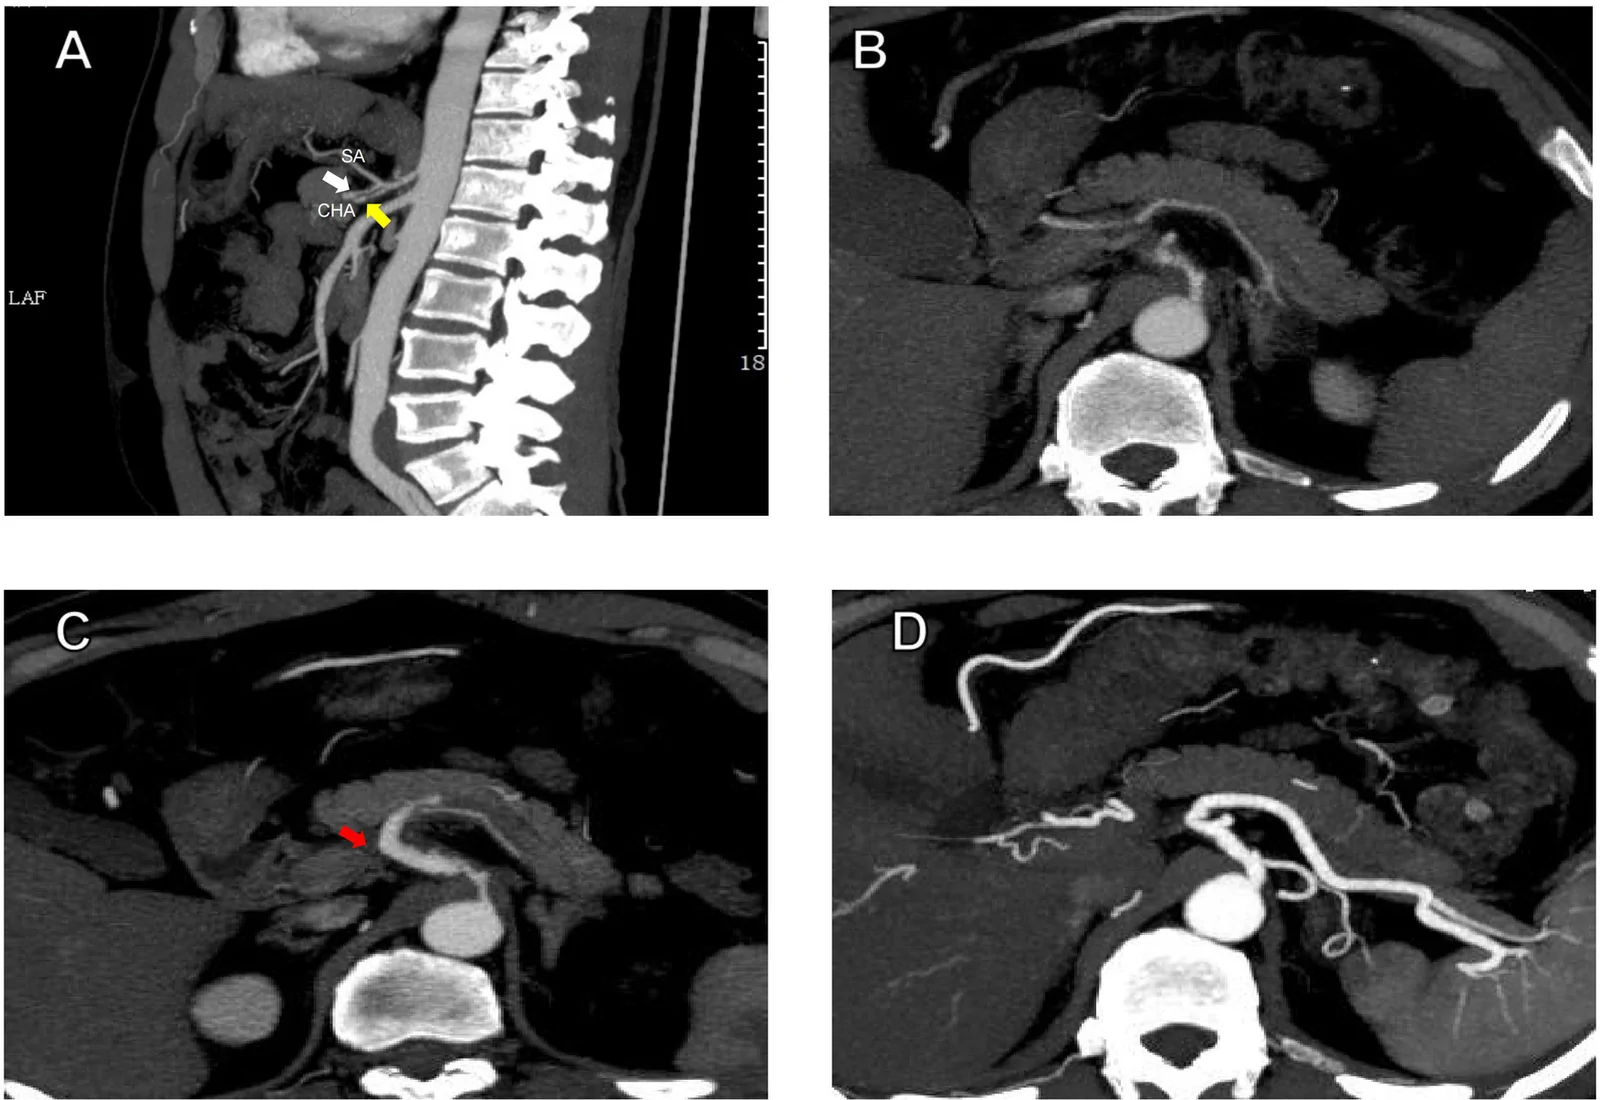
Dissection progression in a conservatively managed patient. (A/B) Pretreatment CT [true lumen (white arrowhead), false lumen (yellow arrow)]. (C) After 5-day conservative management: non-opacification of proximal hepatic artery (red arrow). (D) 6-month follow-up: new collateral circulation in distal hepatic artery. CHA, common hepatic artey; SA, spenic artey.
